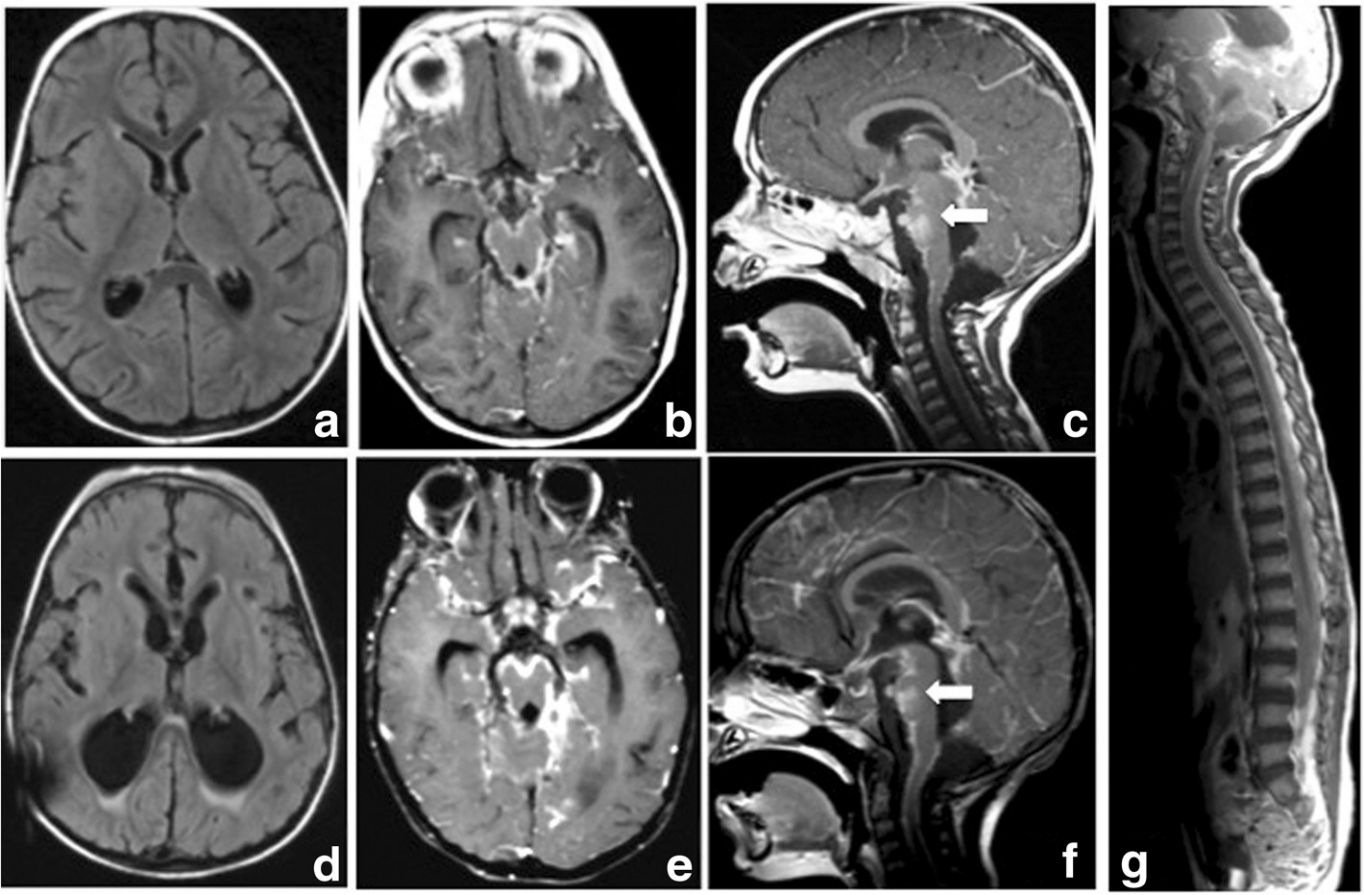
Clinical onset MRI (a, b, c). Follow-up MRI (d, e, f, g). T1 axial basal image (a): no evidence of LMM’s typical hyperintensities. T1 Contrast enhancement images (b, c): intense base and peri-spinal leptomeningeal enhancement and nodular pontine enhancing lesion (white arrow); (e, f, g) increase of enhancing lesions. T1 axial (a, d): progressive hydrocephalus
